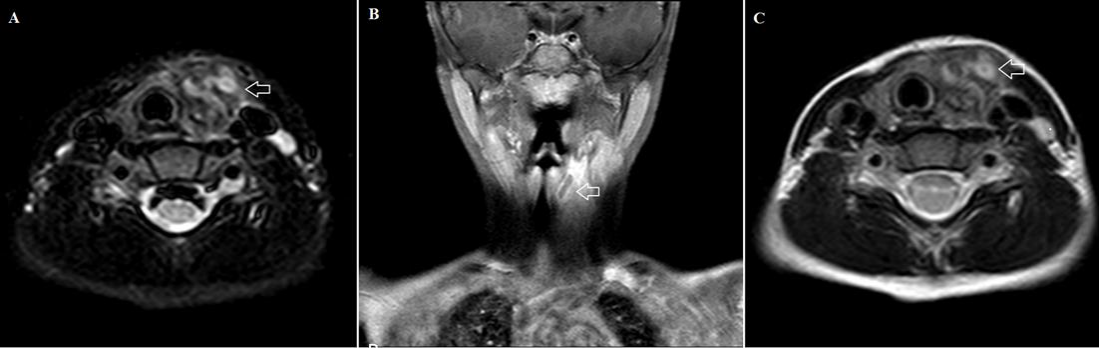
short Tau inversion recovery (STIR), T1-w magnetic resonance imaging (MRI) with contrast administration and T2-w MRI: A) axial STIR image, it is noticed a thin sinus track composed of high signal wall and low signal lumen centrally (air containing); B) coronal T1-w fat sat with paramagnetic iv contrast, the sinus track has distinct enhancing wall, ending near the pharynx; C) axial T2-w image, notice the high signal wall and low signal lumen
